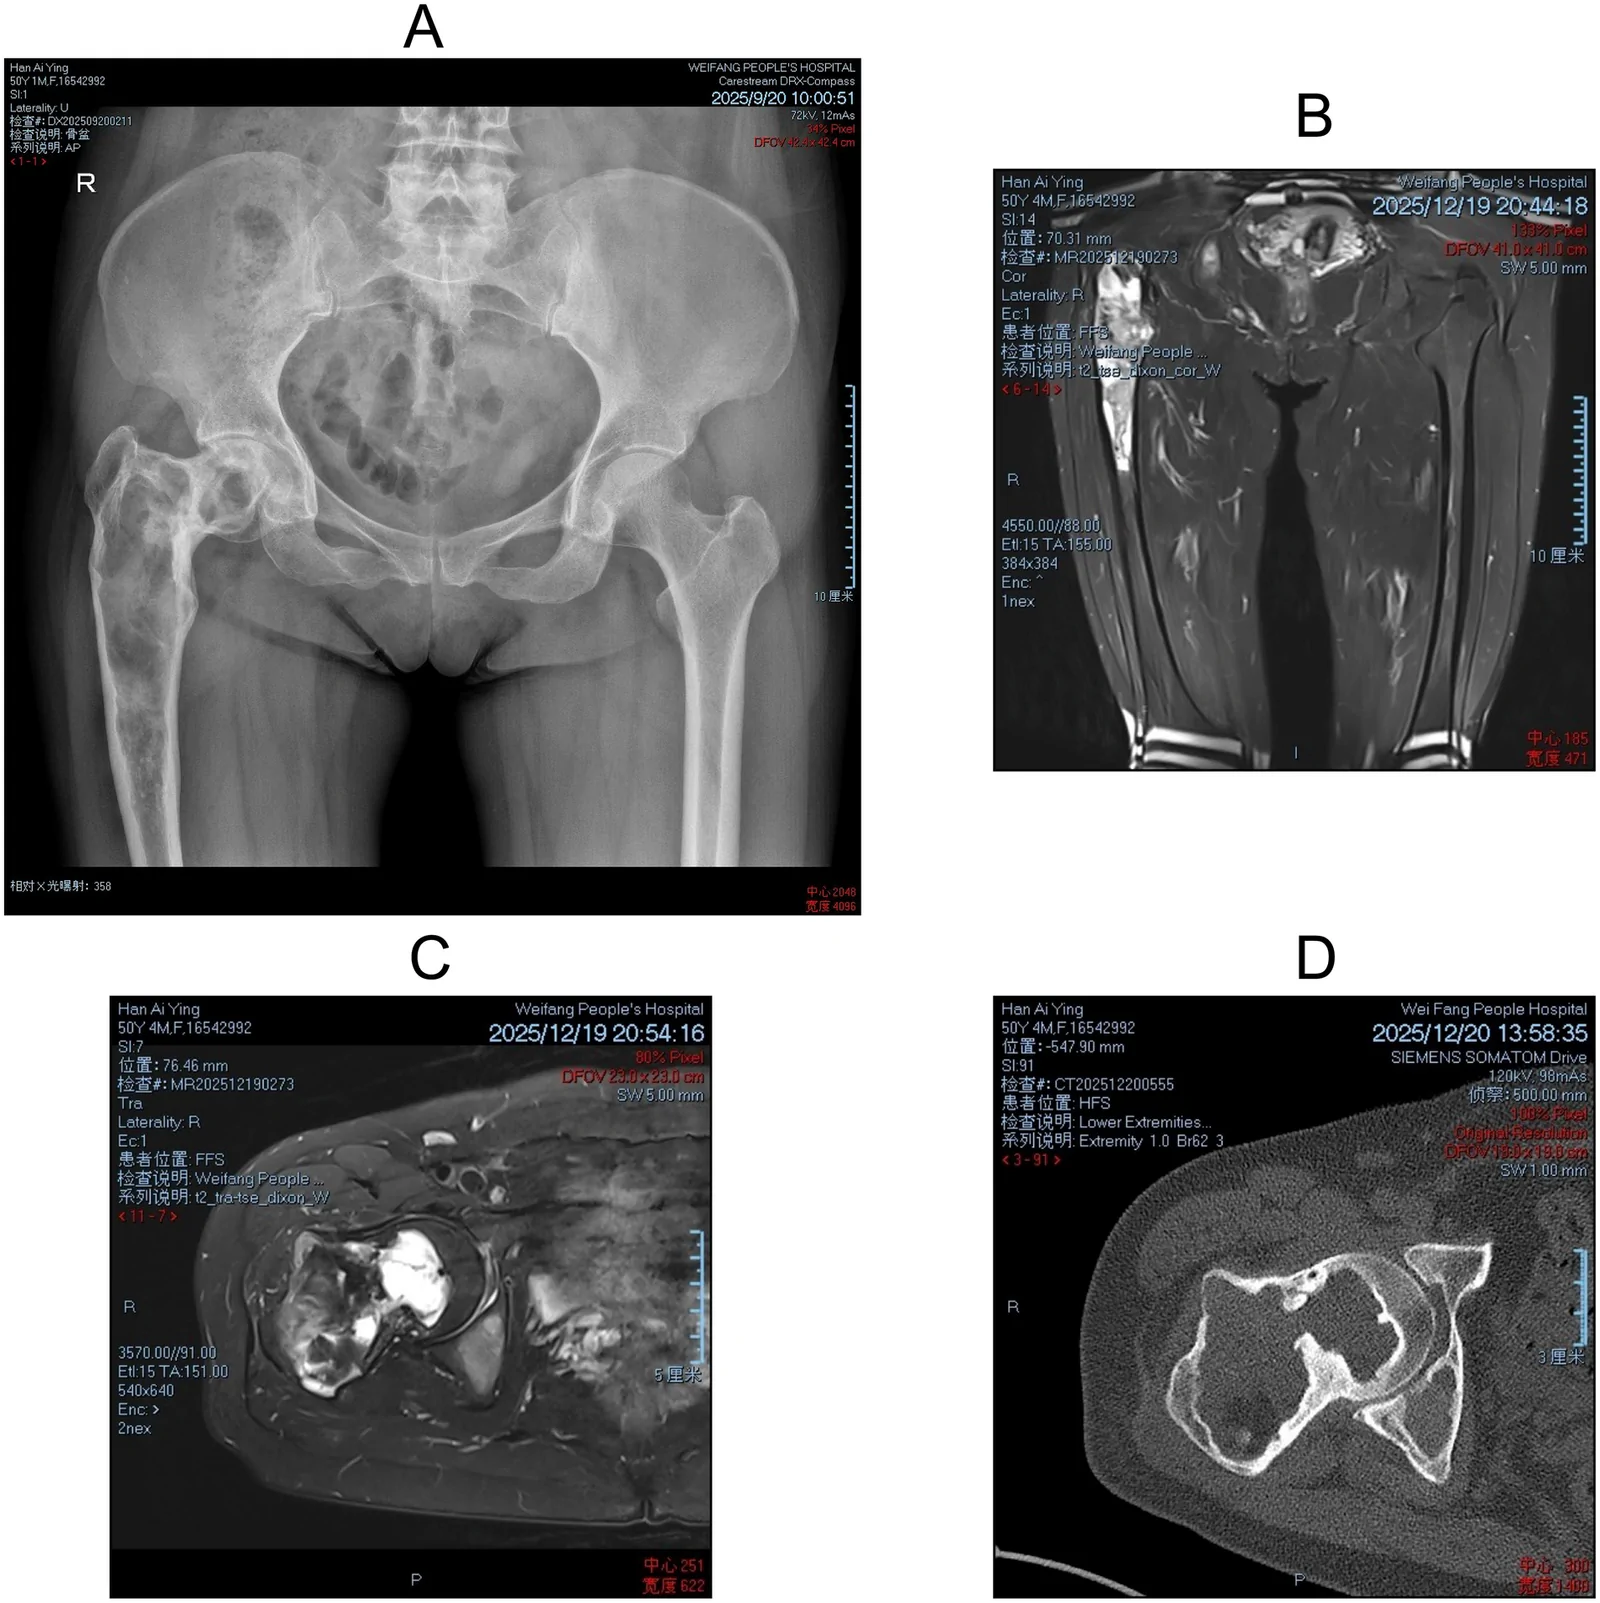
Preoperative imaging. (A) Anteroposterior radiograph of the hip. (B) Coronal MRI of the pelvis. (C) Axial MRI of the right proximal femur. (D) Axial CT scan of the right proximal femur.
